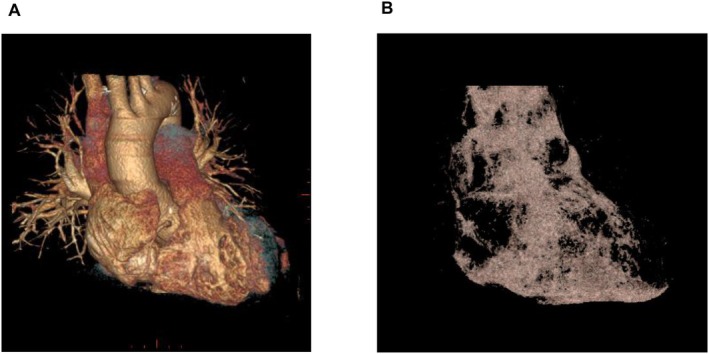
Measurement of pericardial fat (PF) volume. (A) Contrast‐enhanced cardiac computed tomography (CT) with the CT values ranging from −1023 to 3071 HU. (B) Representative constructed CT image with the CT values from −150 to −50 HU indicating PF tissue. HU, Hounsfield unit.
